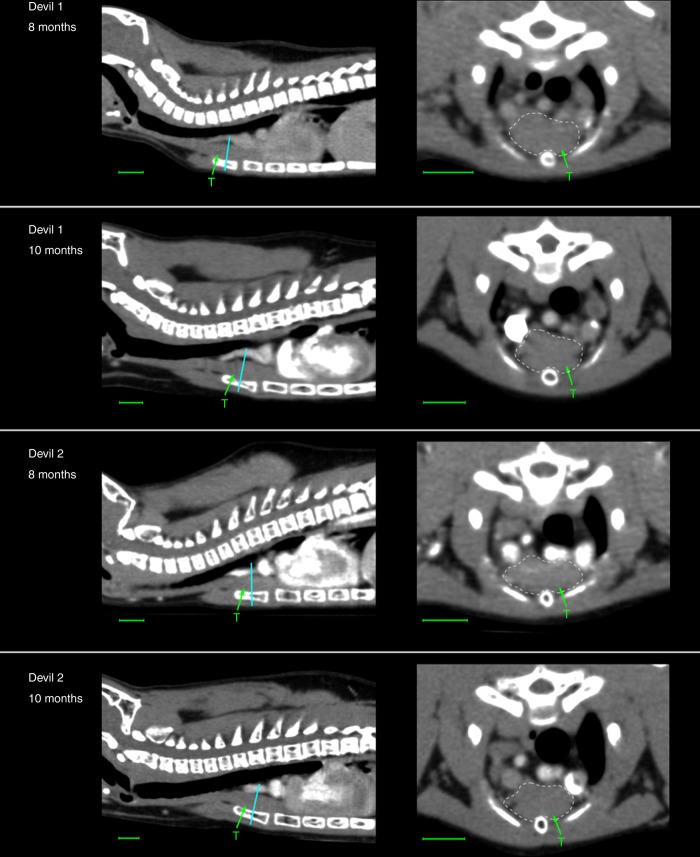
Computed tomography imaging of the thymus (T) in young devils. Cyan lines indicate locations of transverse sections where measurements of the thymus shown in Table 1 were taken. Scale bar under each image represents 1.00 cm
